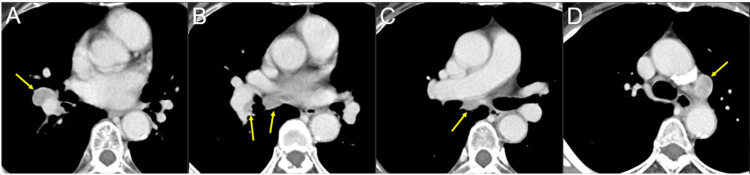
Chest computed tomography images at the initial diagnosis The images show axial CE computed tomography (CT) images (A-D). (A-D) The 2-cm well-demarcated mass lesion in the right middle-inferior perihilar region (arrow in A) and multiple lymph node swelling in the ipsilateral hilar and peribronchial (arrows in B), subcarinal (arrow in C), and subaortic (arrow in D) regions. CE: contrast-enhanced
